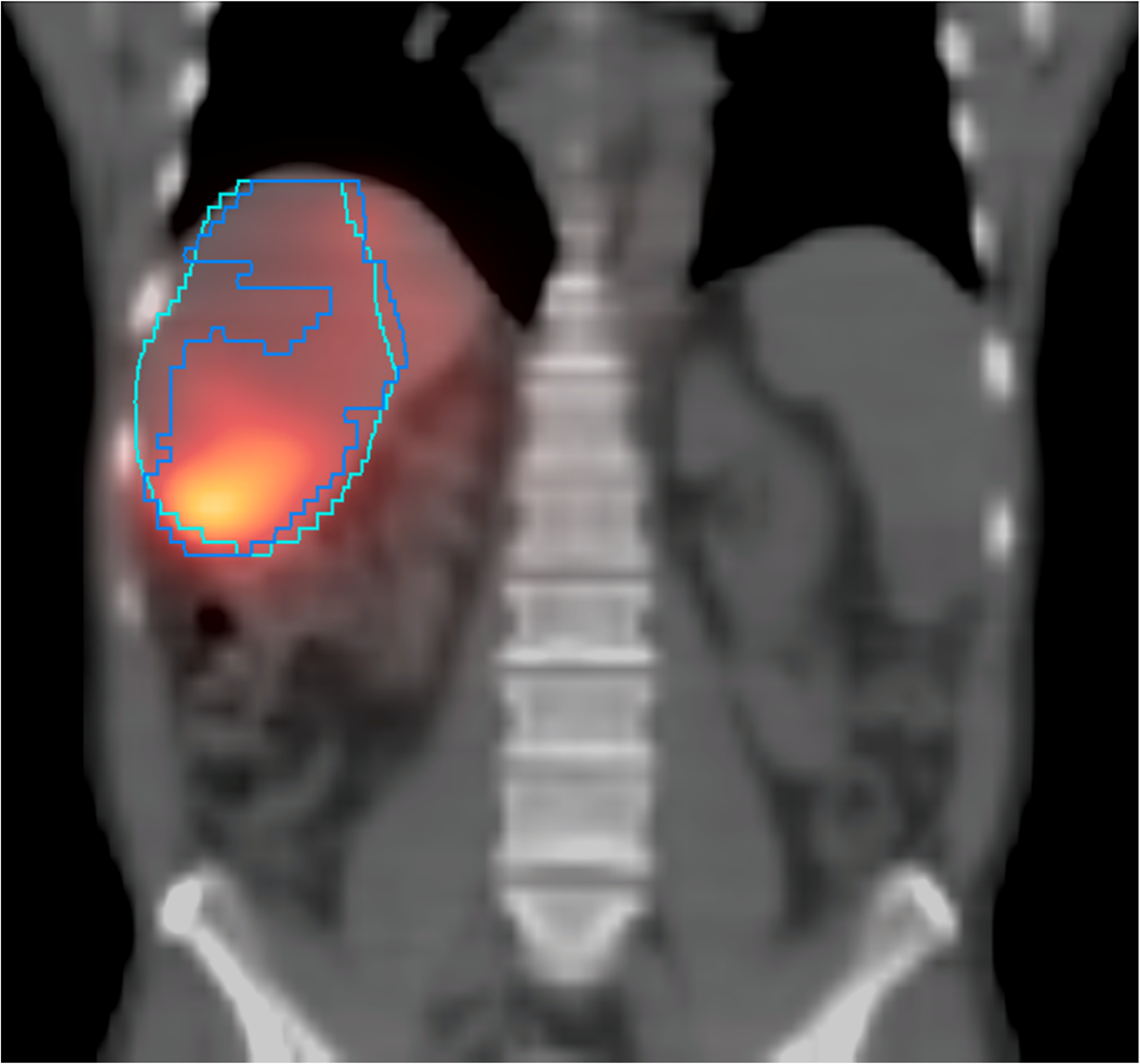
Exemplar case where a VOI delineation based on SPECT thresholding only (blue contour) does not match the CT-based anatomical tumor definition (teal contour). The mismatch results in a difference in tumor volume and mean tumor uptake
